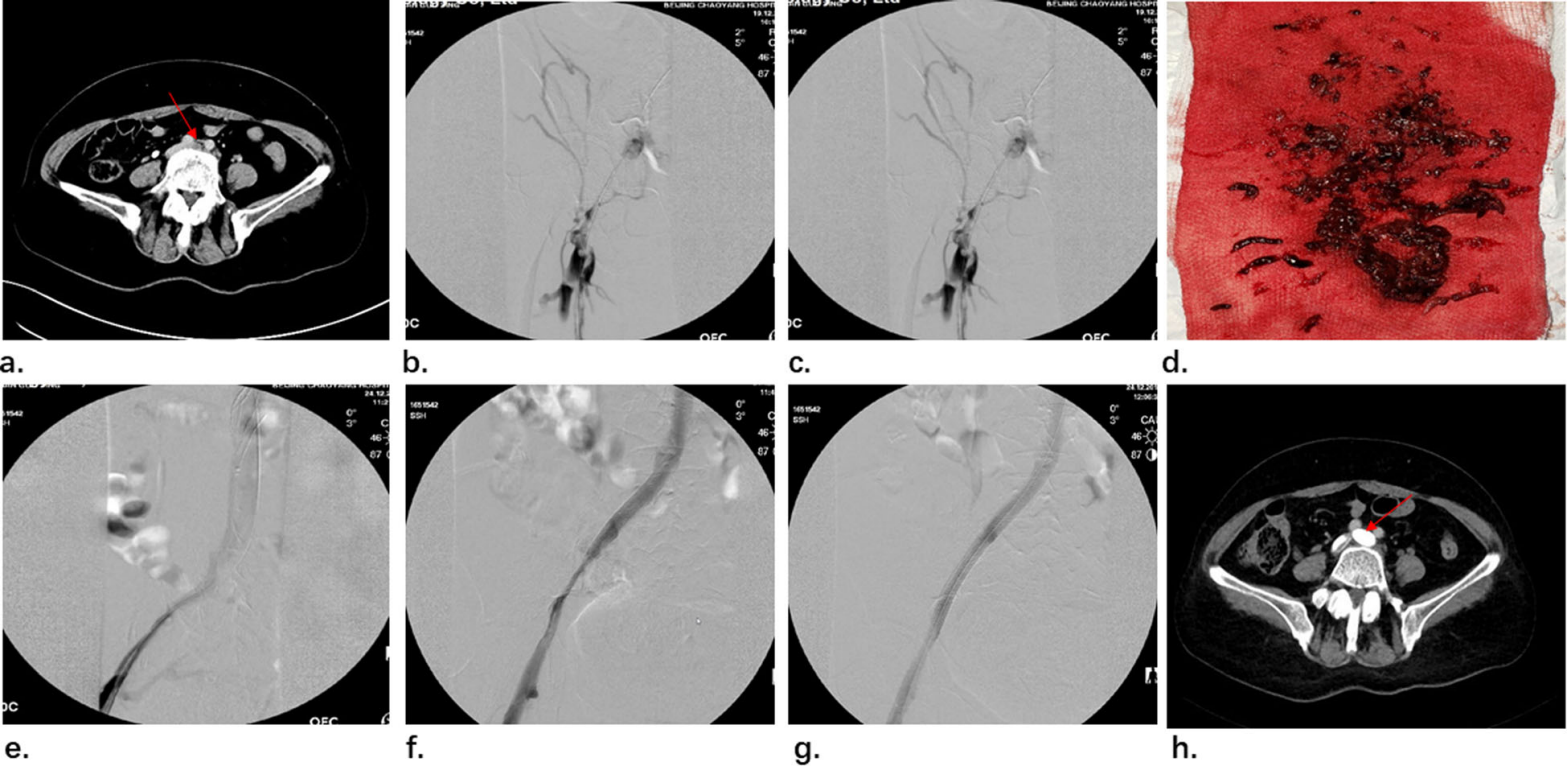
a CT showed May-Thurner syndrome. b the left iliac vein was full of thrombus. c compression and occlusion of the left common iliac vein were observed after thrombus aspiration (d) the blood clot that was aspirated out (e) compression of the left common iliac vein were showed after catheter thrombolysis and expansion by 12 mm*40 mm XXL balloon. f the first stent. g the second stent. h CT image of left iliac vein after 1 year
